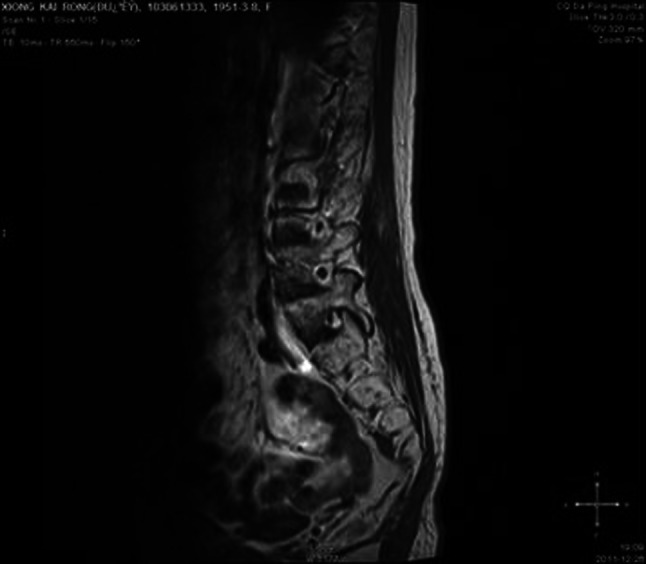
Magnetic resonance imaging (MRI) scan. MRI prompted the vertebral bone destruction
